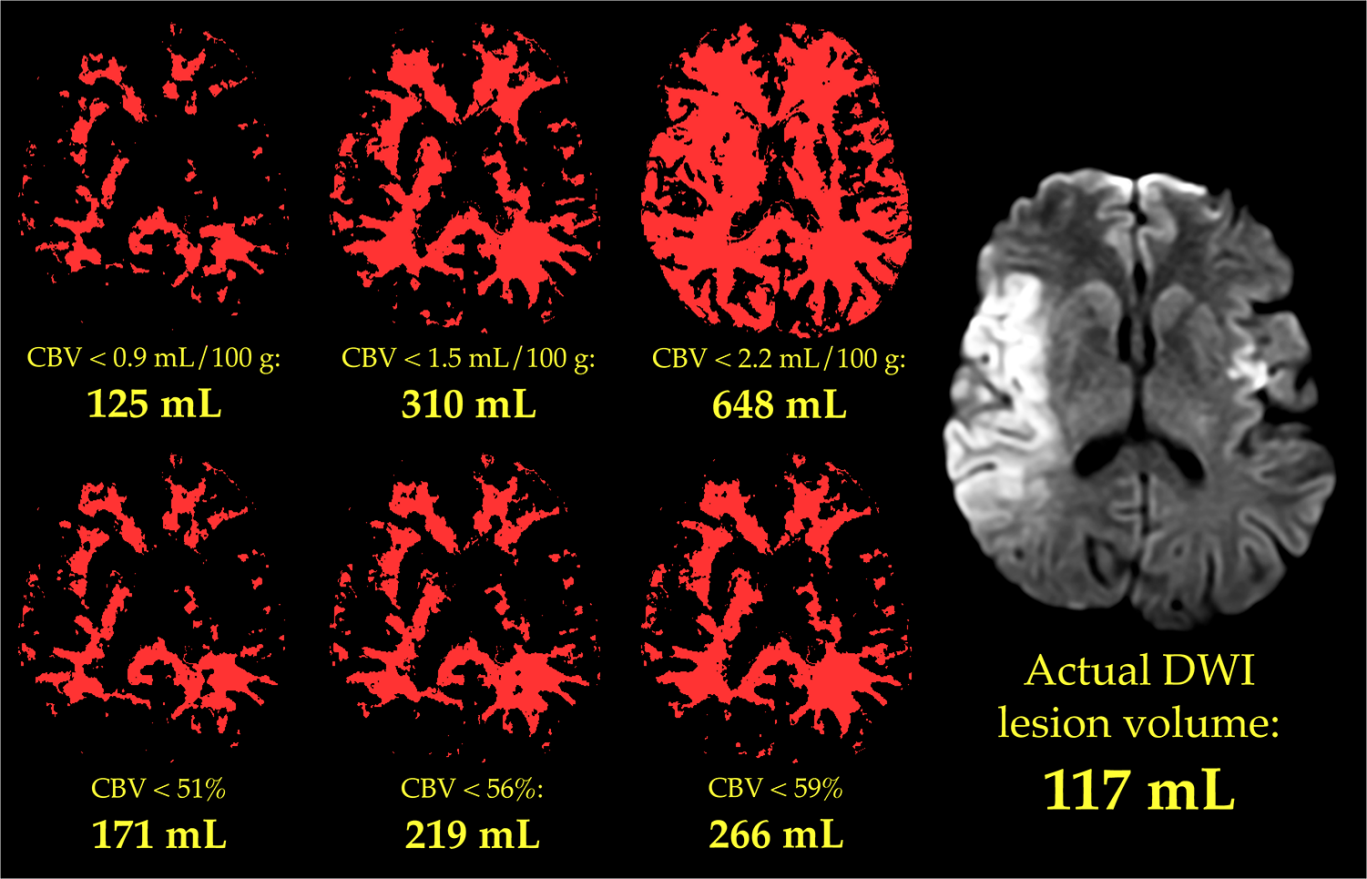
Objective measurement of CBV lesion volume. The six smaller images depict low-CBV lesions that were delineated automatically, in a single slice of one patient’s CBV maps, by rendering in white all brain pixels in which CBV was below each of six different thresholds. Each threshold is listed below its corresponding image, along with the total volume of tissue with CBV below that threshold in all slices. For clarity, the lesions identified by only three of the six absolute thresholds are shown in the top row, and lesions identified by only three of the six relative thresholds are shown in the bottom row. The larger image on the right is a DWI image obtained from the same patient, at approximately the same level. The gold standard core volume, measured over all DWI slices, was 117 mL.
